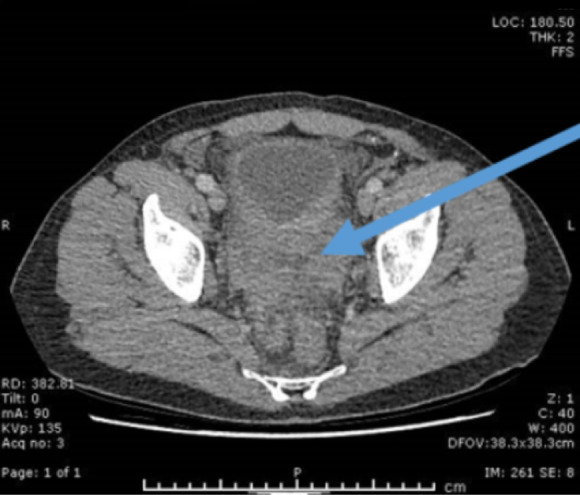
Cross-sectional CT image showing abnormal bladder suspicious of either an inflammatory or malignant process. CT, computed tomography.
